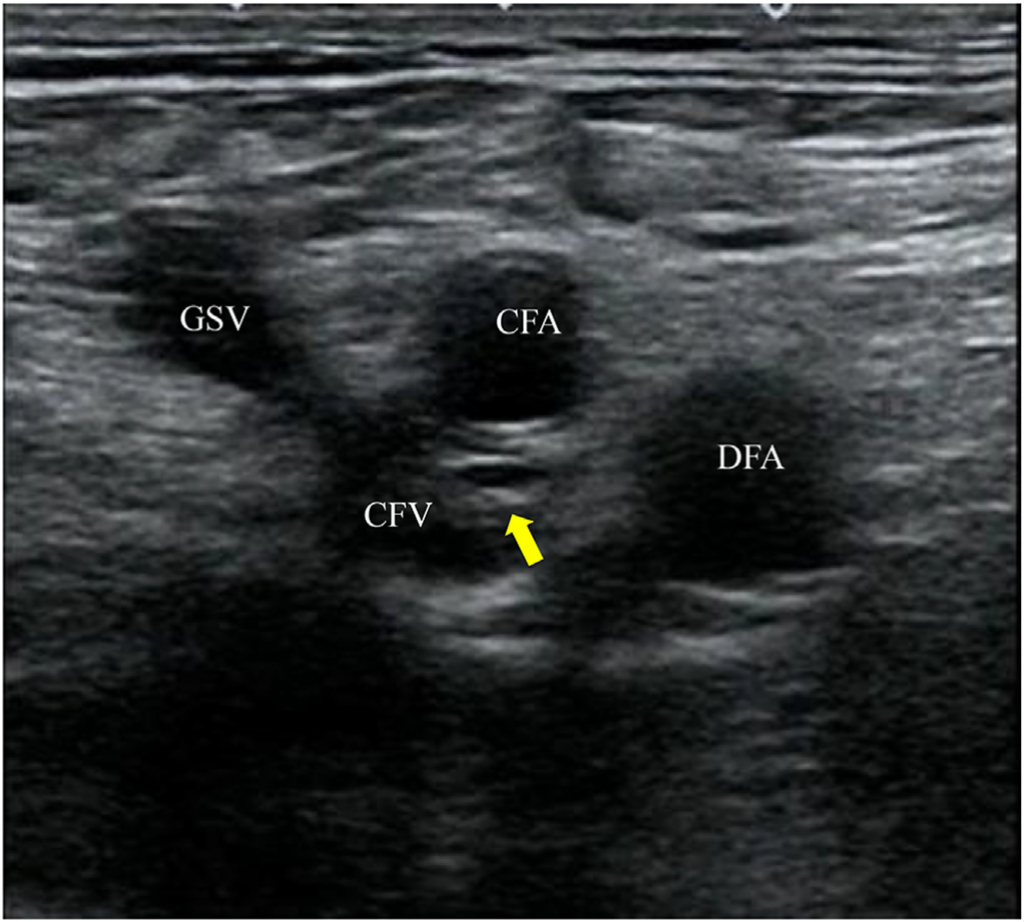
Ultrasound images showing post-thrombotic changes in the common femoral vein (CFV). Trabeculae in the CFV are observed below the saphenofemoral junction (SFJ) and above the deep femoral vein (DFV) bifurcation. *CFA,* Common femoral artery; *DFA,* deep femoral artery; *GSV,* great saphenous vein.
